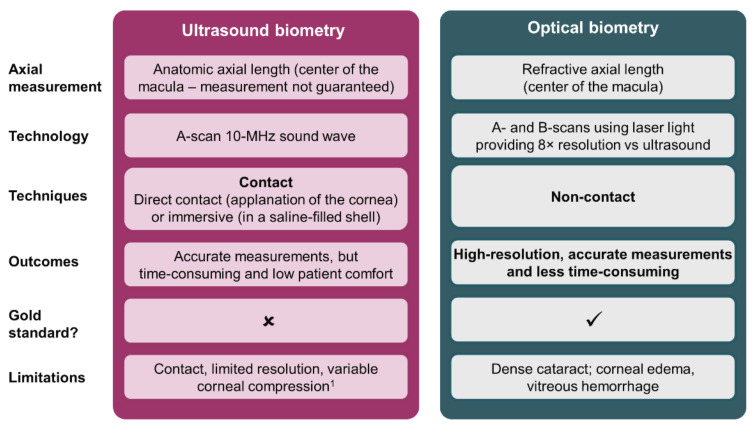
Overview of ultrasound and optical biometry techniques [5,8,9,17,18,19]. ^1^ Only applicable to applanation biometry, corneal compression is not observed in immersion biometry.
